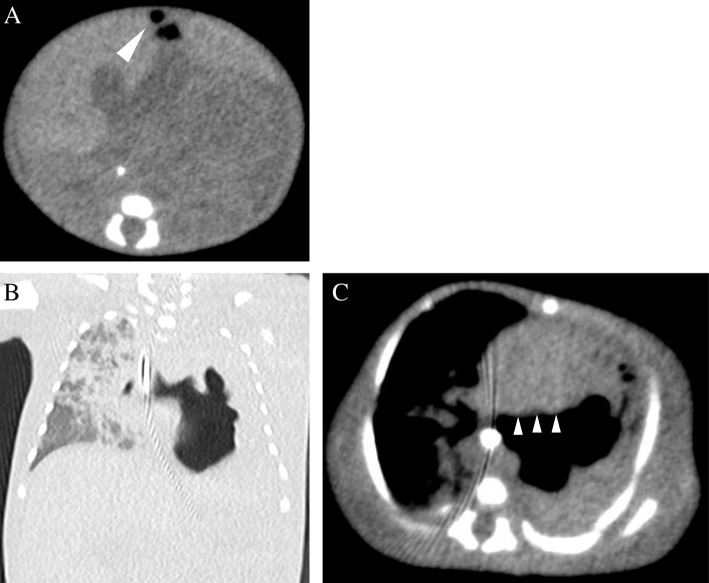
Computed tomography. a Mediastinal-conditional computed tomography image showing a small amount of free air (arrowhead). b Lung-conditional computed tomography image showing a giant bulla in the left lower lung field. c Mediastinal-conditional computed tomography image showing that this bulla was compressing the extrinsic pulmonary vein and left atrium from the dorsal side
